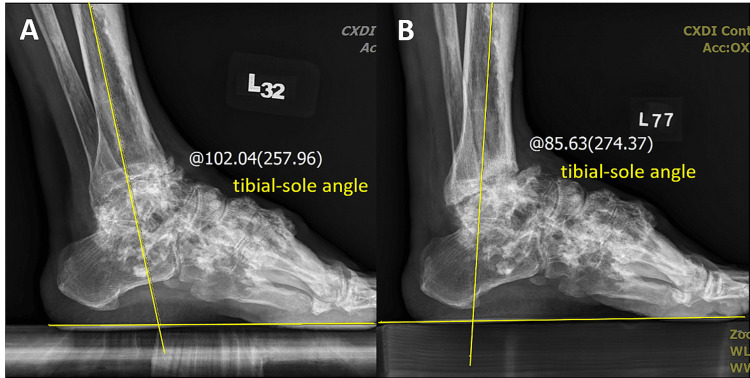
The tibial-sole angle was corrected from preoperative 102.04° to postoperative 85.63° in the lateral view of ankle weight-bearing radiographs. Preoperative (A) and postoperative (B) images.
